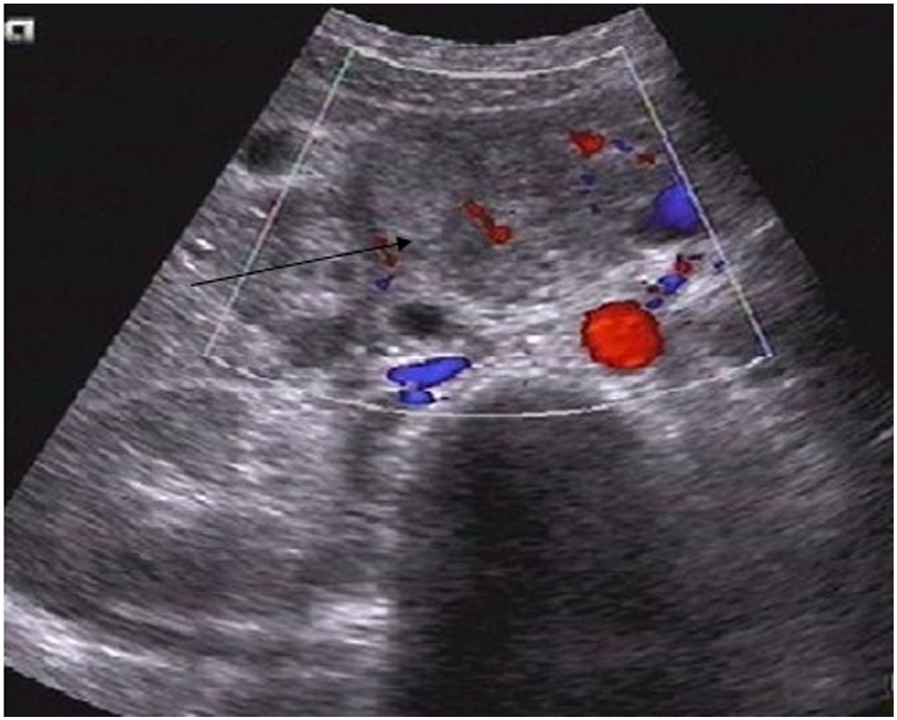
Sonography revealed a 7.1 cm × 7.2 cm hypoechoic mass at the wall of the duodenum and pancreas (arrow).
